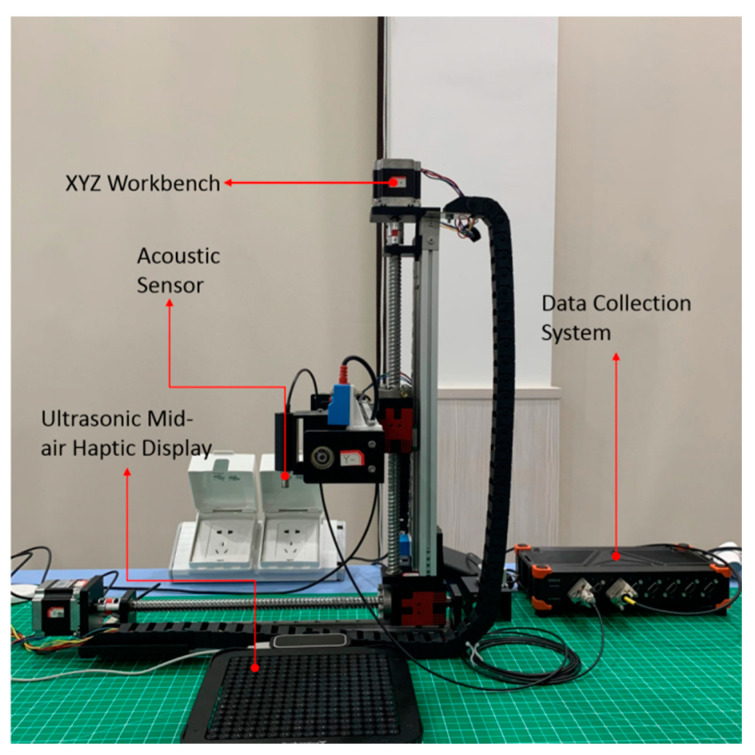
Measurement setup: XYZ workbench, data collector, acoustic sensor, ultrasonic mid-air haptic display.
